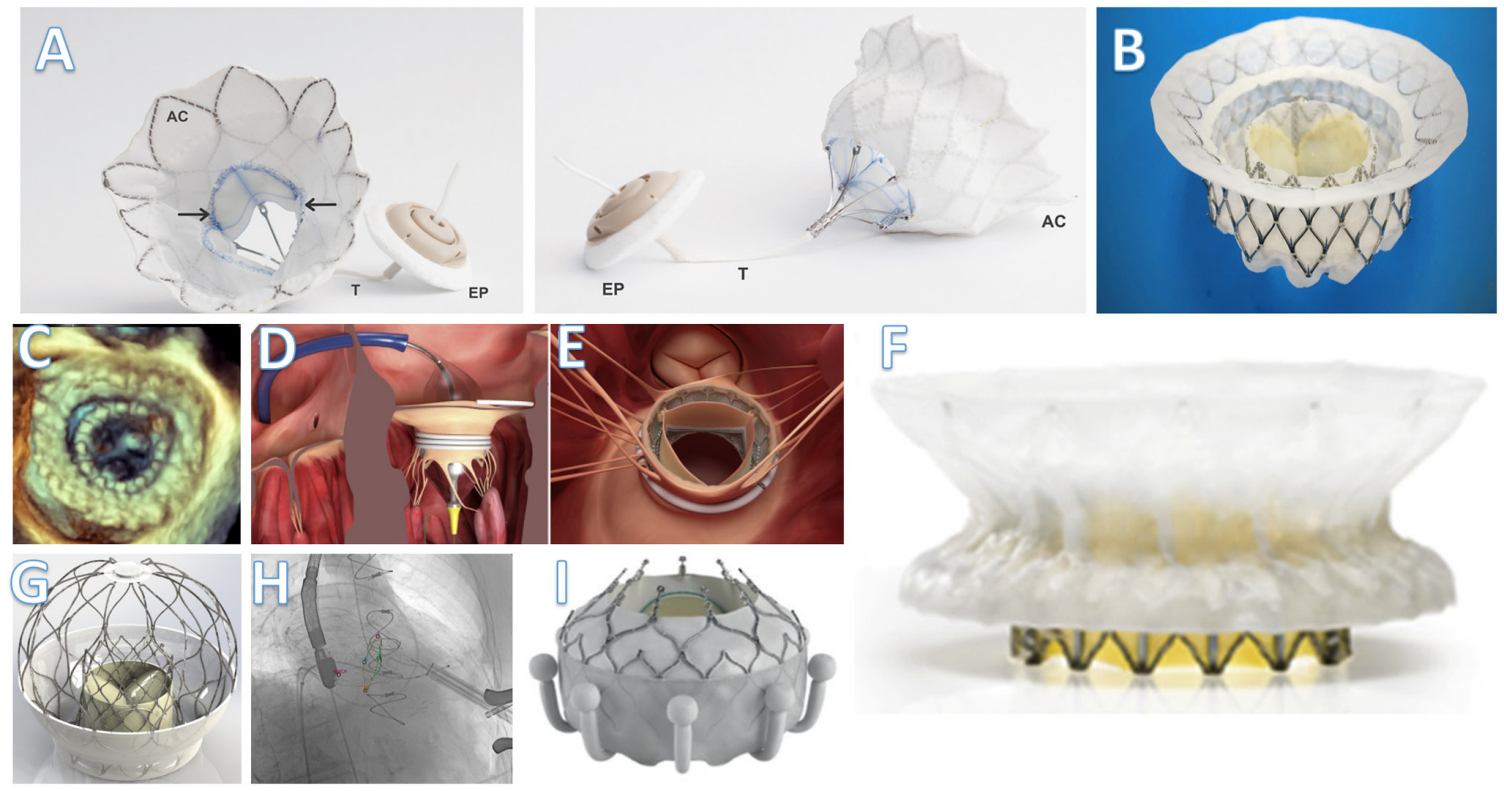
Transapical and transseptal transcatheter mitral valve replacement (TMVR) systems. (A) Tendyne valve, courtesy of Abbott; (B) Intrepid valve, courtesy of Medtronic; (C) three-dimensional transesophageal echocardiogram showing the Intrepid valve after implantation; (D) Sapien M3 valve, transseptal placement of the docking system (both D and E permission obtained from Elsevier; (E) surgeon’s view of the Sapien M3 after placement; (F) Cephea valve, courtesy of Abbott; (G) AltaValve, courtesy of 4CMedical; (H) fluoroscopic appearance of the AltaValve during TMVR; and (I) Evoque valve, courtesy of Edwards Lifesciences. AC: outer frame with a cuff; EP: epicardial pad; T: Tether. From Aoun J et al. Transcatheter mitral valve replacement: an update. Curr Opin Cardiol. 2021 Jul;36(4):384-89. Copyright ©2021 Wolters Kluwer Health, Inc. All rights reserved.
